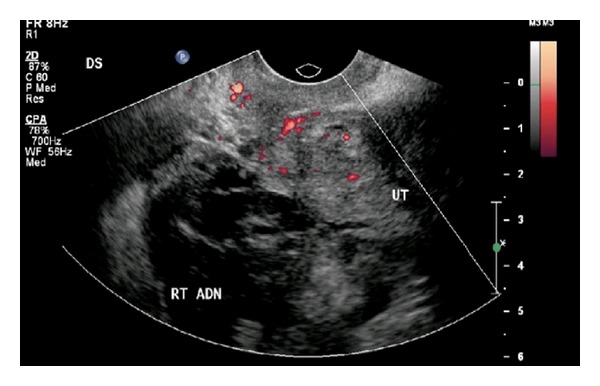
Pelvic ultrasound demonstrates a right adnexal mass (labeled RT ADN) adjacent to the uterus (labeled UT) with surrounding hypoechoic fluid suspicious for ectopic pregnancy.
